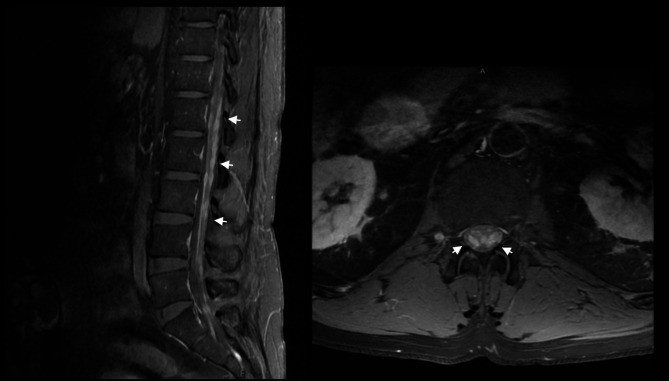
Sagittal (left) and axial (right) T1-weighted post-gadolinium magnetic resonance imaging sequences demonstrating prominent nodular leptomeningeal deposits (arrows) surrounding the conus medullaris and involving the cauda equina nerve roots
